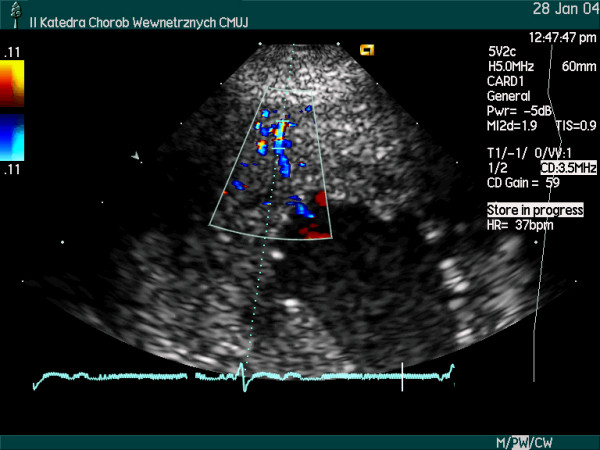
The orientation of Doppler gate within the LAD perforator.
